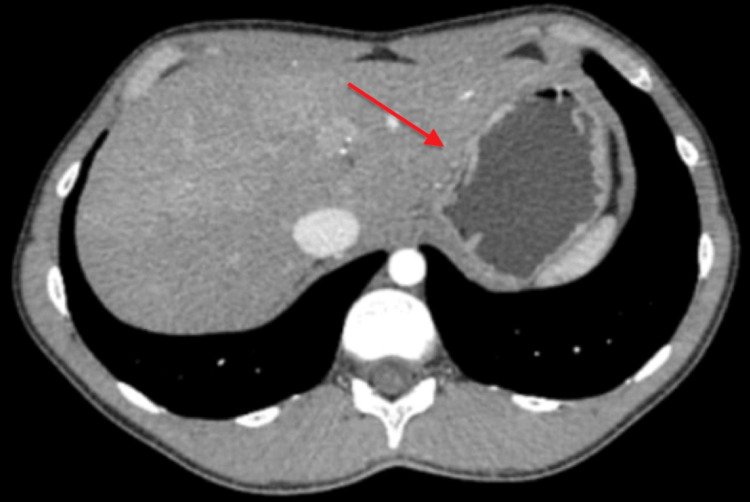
Abdominal contrast-enhanced CT showing a large, heterogeneous, lobulated, well-defined mass, apparently originating from the IV B segment, the V segment, and a portion of the VI segment of the liver, as indicated by the red arrow.
